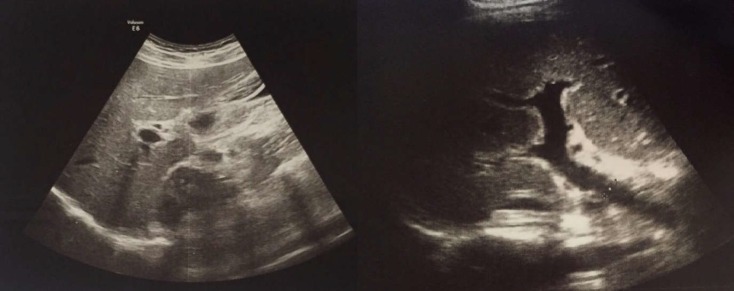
Sonography imaging of the liver
